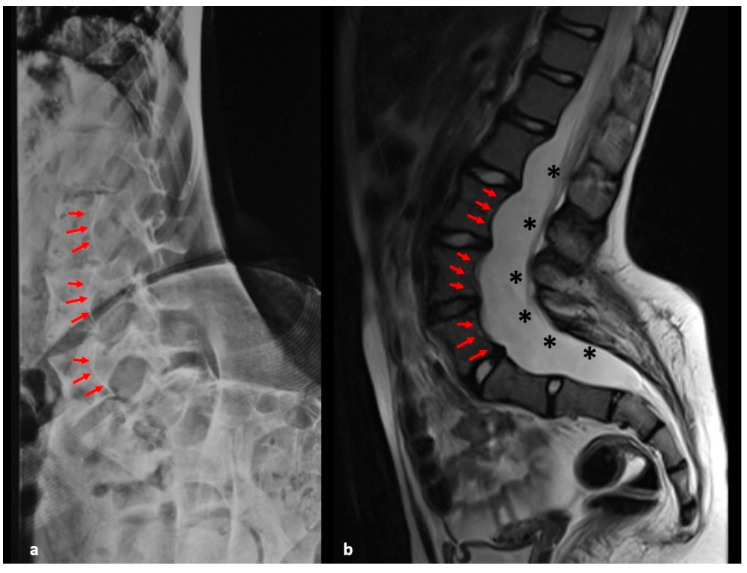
X-ray (a) and MRI (b) of the lumbar spine of a child (F) with NF1 and dystrophic scoliosis. Note the vertebral scalloping (arrows) and the dural ectasia (asterisks).
